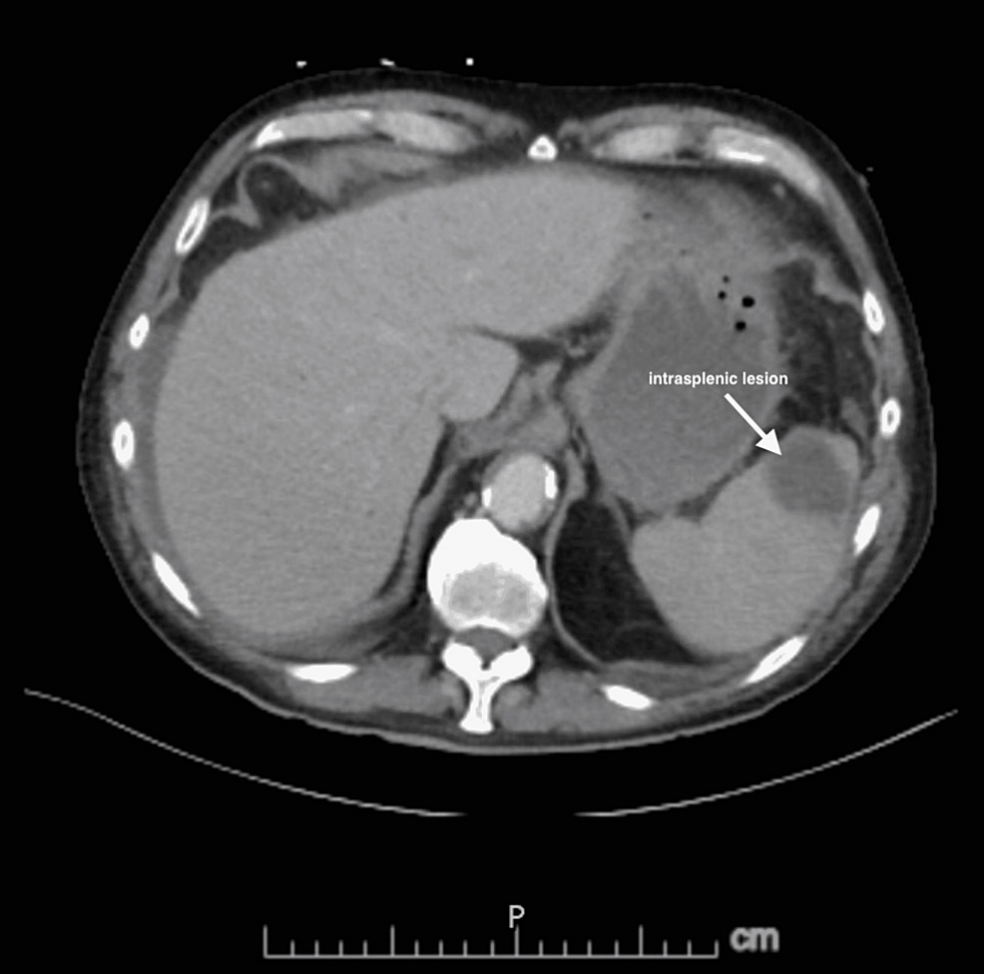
Abdominal CT scan showing an intrasplenic lesion.
